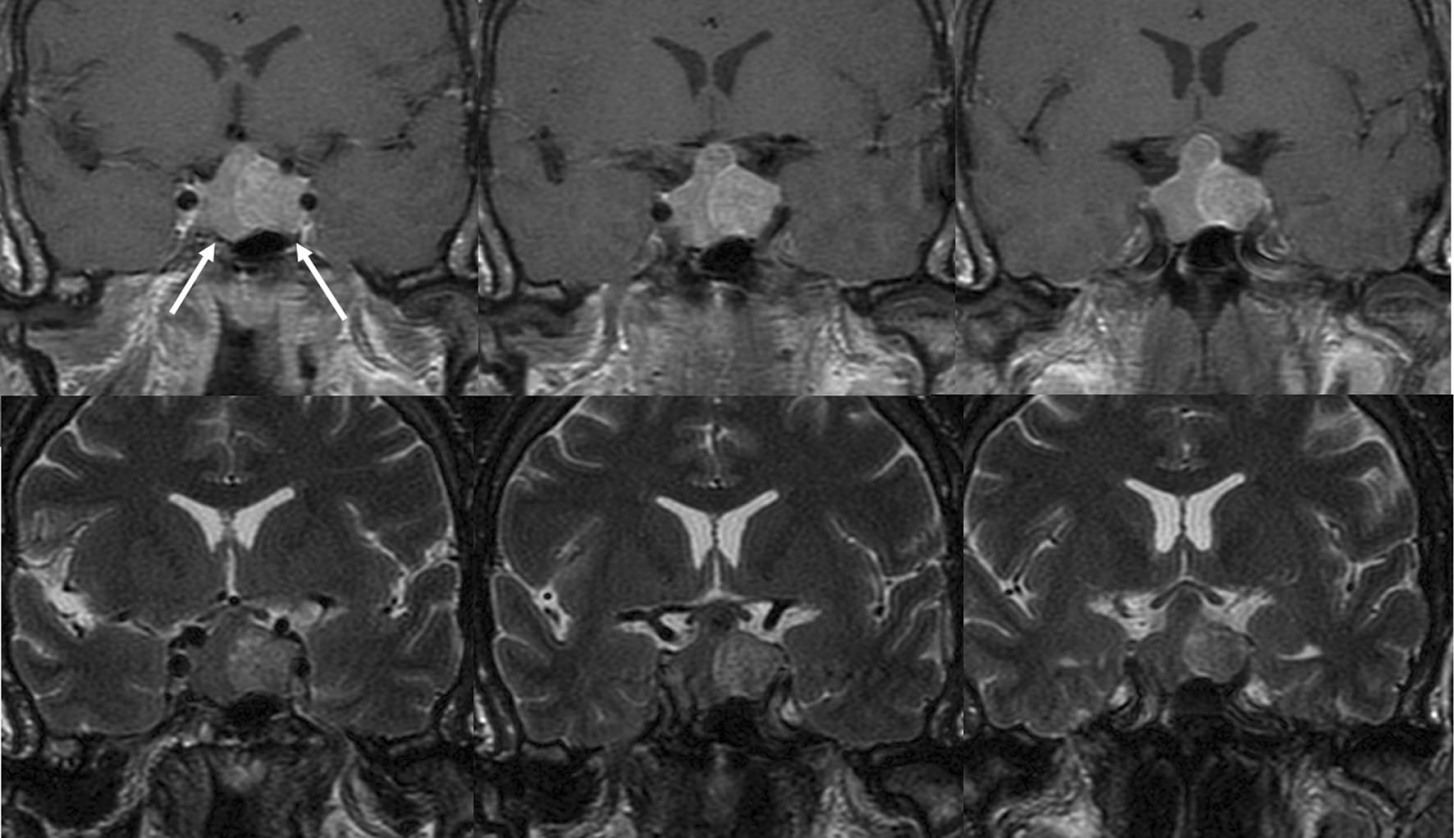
Case no. 1, coronal T1 (upper row) and coronal T2 (lower row) weighted MR imaging identified two clearly separate pituitary macroadenomas with different signal intensity. Left and right arrows indicate respectively somatotroph and gonadotroph tumors. Both tumors were separated by flattened pituitary gland (visible on T1 weighted MR imaging as a hyperintensive band between the two tumors and confirmed during transsphenoidal operation)
